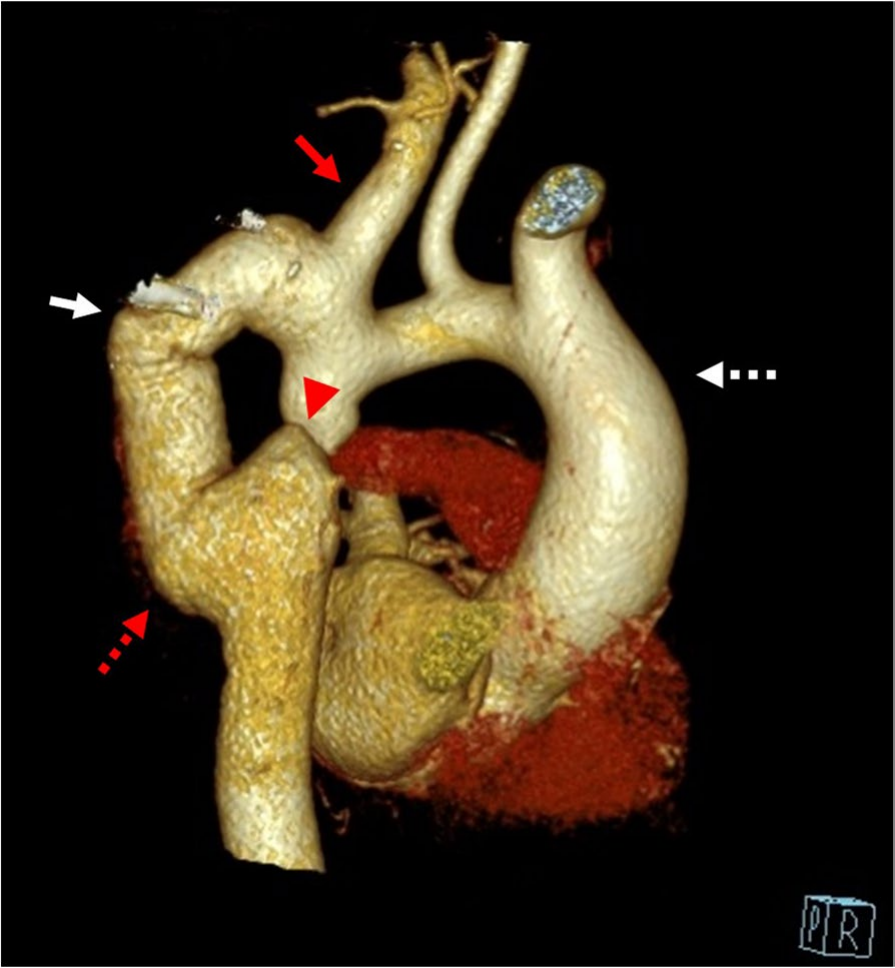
CT angiography of thorasic aorta (3D reconstruction). Red Arrow:the left Subclavian artery is ectatic, reflecting a more diffuse arteriopathy, Arrow head: Aortic interruption, Red Dashed arrow: pseudoaneurysm of distal anastomotic site, White arrow: pseudoaneurysm of mid posterior part of the tube graft. White Dashed Arrow: Ascending Aorta
